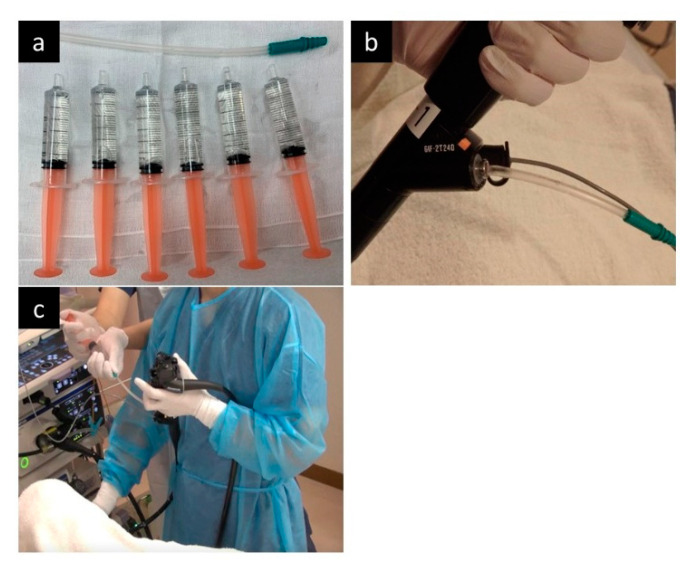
The jelly-filling method in the endoscopic ultrasonography (EUS). (a) Jellies is prepared in 5-mL syringes, and a short 14Fr catheter is also prepared. (b) After inserting the endoscope, insert the 14Fr catheter through the wide forceps channel and insert the ultrasound probe through the other forceps channel. (c) During EUS observation, additional jelly injections are performed by the assistant, not the operator.
